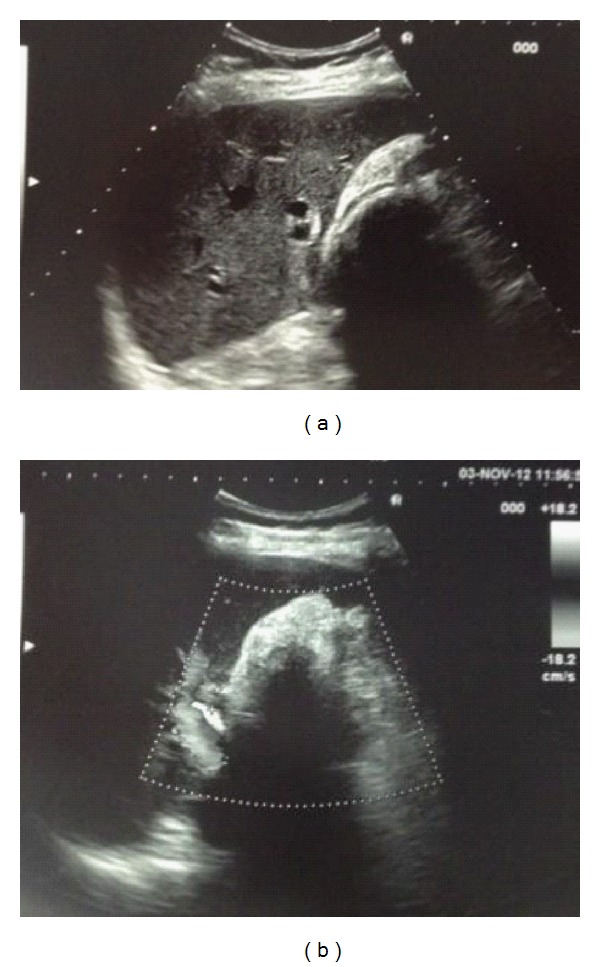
Abdominal ultrasound showing a gallbladder with thick walls and one large gallstone (50 mm) entrapped into the Hartmann pouch.
